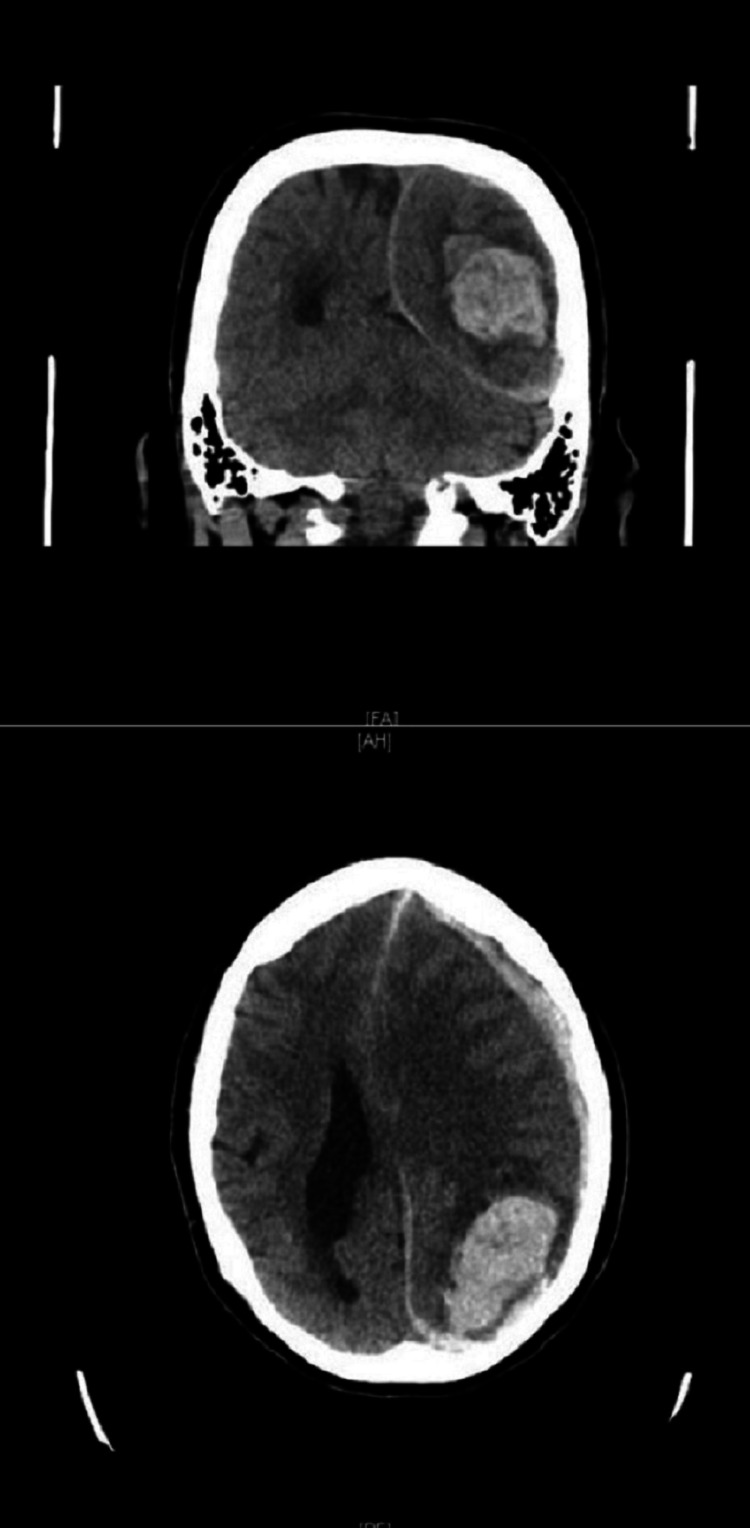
CT Scan of the Head CT scan of the head obtained on presentation in the emergency department, notable for left posterior intracranial hemorrhage. CT, computed tomography.
